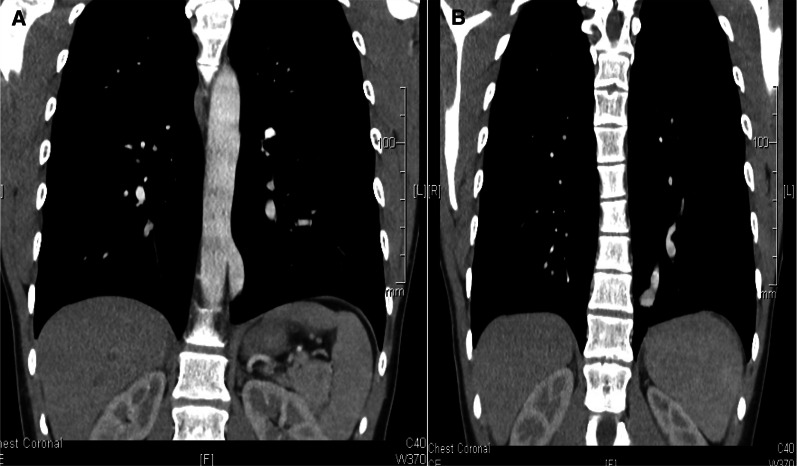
A, B Coronal CT views showing systemic arterial supply taking origin from the DTA
